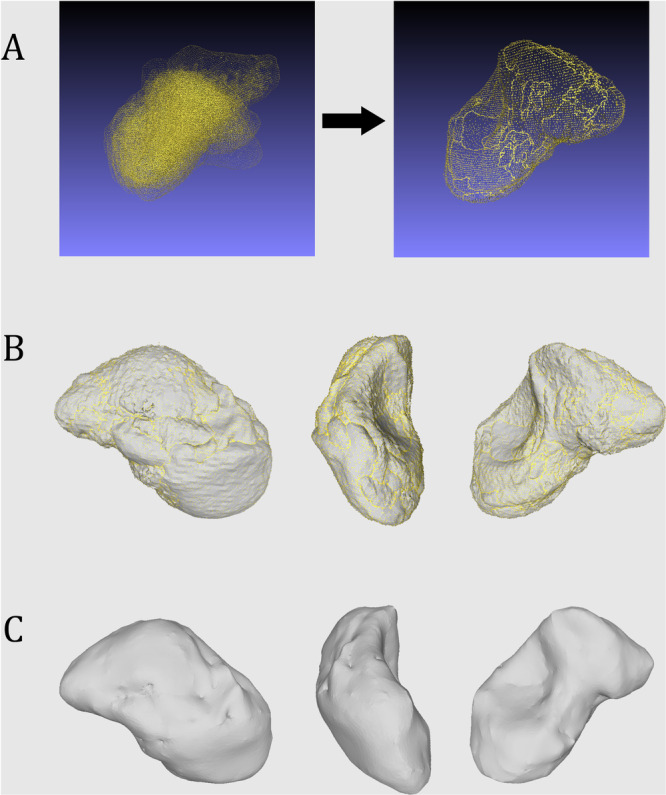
Generation of the mean 3D shape model of a scaphoid. (A) Cloud representation of multiple 3D surface scaphoid model before and after manual alignment and Boolean fusion, based on scaphoid CT of 17 patients. (B) Radial, dorsal and ulnar view of 3D surface scaphoid model after fusion. (C) Final model after edge smoothing.
